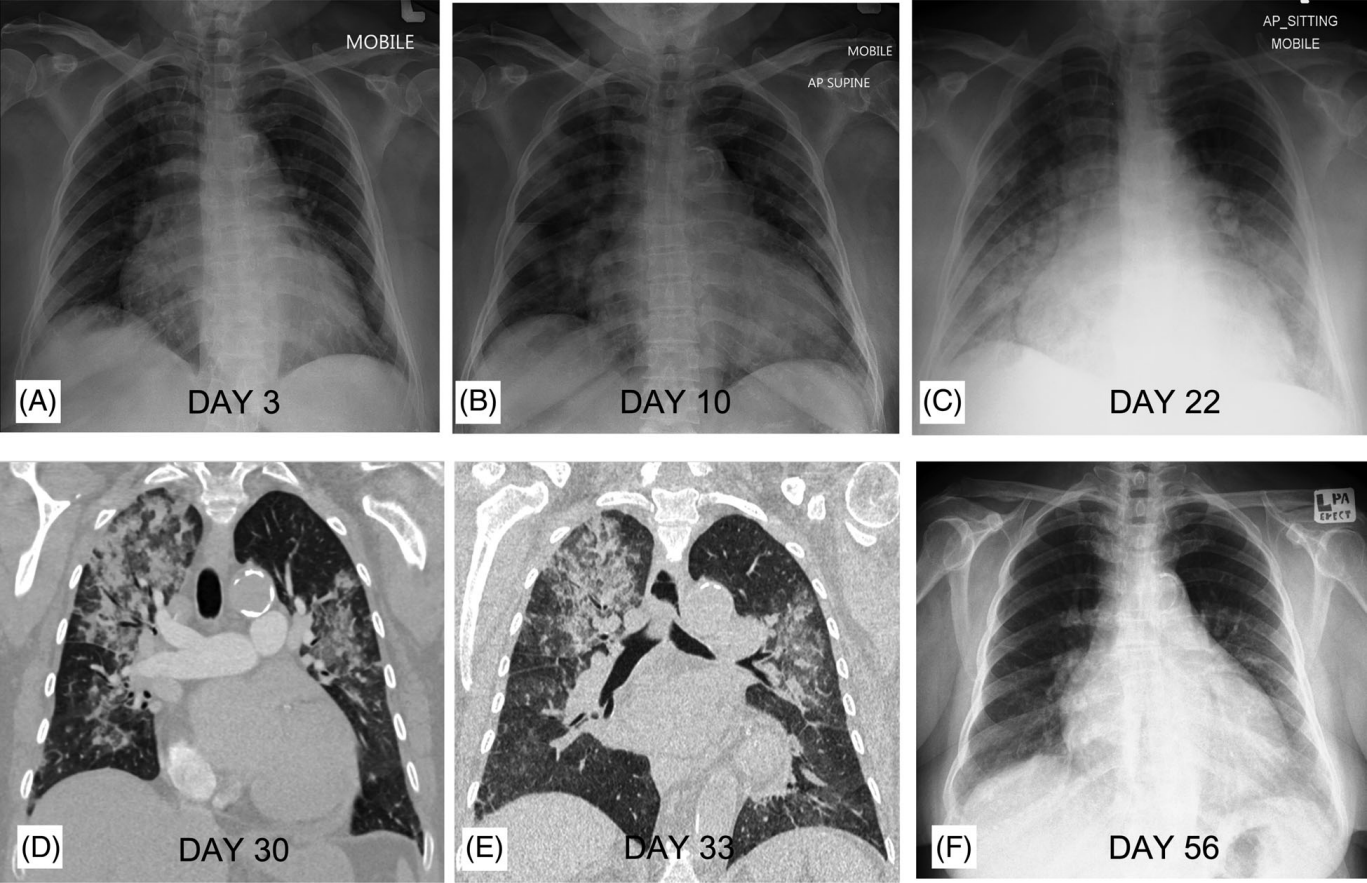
Chest X‐ray (CXR; A–C and F) and coronal reconstruction of computed tomography (CT) in lung window (D, E). (A) Day 3 of coronavirus disease 2019 (COVID‐19) diagnosis: no evidence of lung consolidation. (B) Day 10: bilateral peripheral consolidations. (C) Day 22: bilateral mid to lower zone consolidations which appeared more confluent. (D) Day 30: perilobular consolidations surrounding ground‐glass opacities in keeping with organizing pneumonia (OP). (E) Day 33: OP started to improve following recommencement of steroid therapy. (F) Day 56: resolved lung changes.
